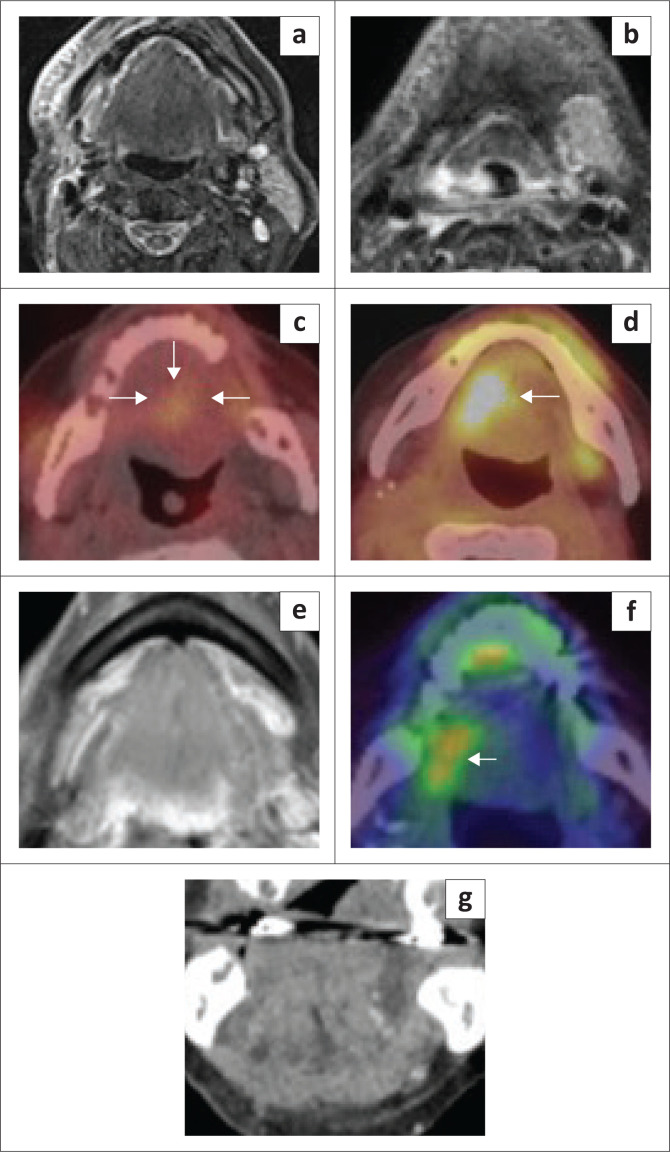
False-positive uptake on positron emission tomography (PET) imaging. An elderly female patient who underwent resection of an oral tongue squamous cell carcinoma and neck dissection, followed by radiotherapy. The baseline post-treatment T2 axial images (a, b) show marked oedema in the right upper neck and floor of the mouth (FOM) region with an absent right submandibular gland and oedematous left submandibular gland. A ^18^F-fluorodeoxyglucose positron emission tomography/computed tomography (^18^F-FDG PET/CT) (c) performed after 6 months for a synchronous sinister lung lesion showed non-significant uptake at the FOM. A follow-up ^18^F-FDG PET/CT (d) performed for a malignant lung lesion after 1 year showed significant uptake at the right FOM; however, the MRI (e, post-contrast fat-suppressed axial) and clinical examination did not reveal any mass lesion at the FOM. Persistent PET uptake after another year (f) and normal FOM on coronal contrast-enhanced CT neck (g).
